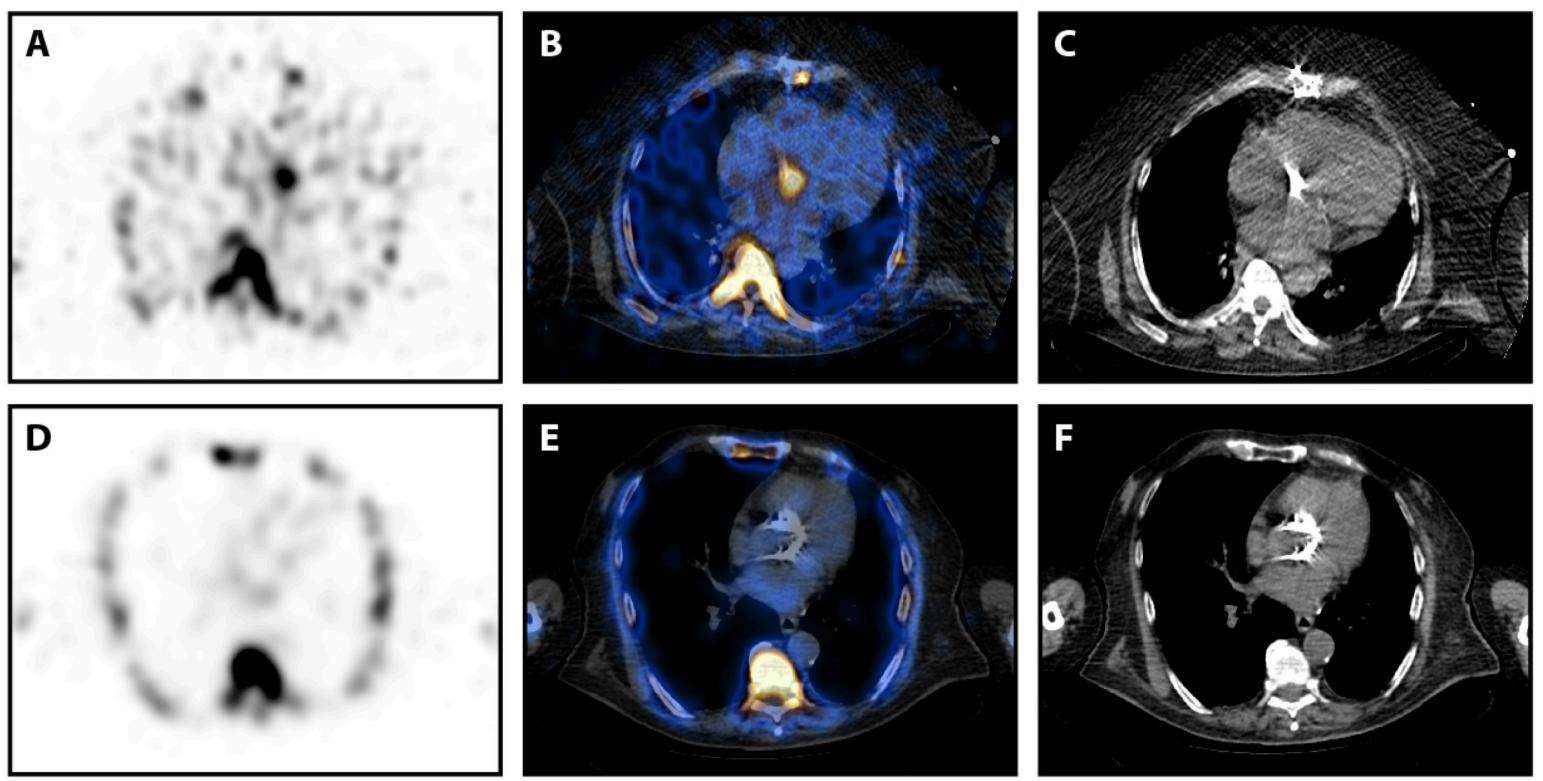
^99m^Tc-Besilesomab-SPECT/CT in patients with aortic valve prostheses. (A–C) SPECT/CT images in transversal view of a 63-years old male patient with suspected prosthetic valve infection 5 years after an aortic valve replacement. Blood cultures detected *Staphylococcus aureus* and TEE suspected an abscess formation. SPECT showed a distinct focal uptake at the aortic valve level (A). This focal uptake could be localized around the prosthetic valve in fused images (B). (C) Non-fused CT image of the prosthetic valve. Pathology results confirmed infection of the prosthesis. (D,E) SPECT/CT images in transversal view of an 82-years old male patient with suspected prosthetic valve infection 3 years after a transcatheter aortic valve implantation. Blood cultures were negative and TEE was inconclusive. SPECT did not detect any pathological uptake (D), especially around the aortic valve replacement (E). (F) Non-fused CT image of the prosthetic valve. Endocarditis was excluded by clinical findings according to the Duke criteria.
